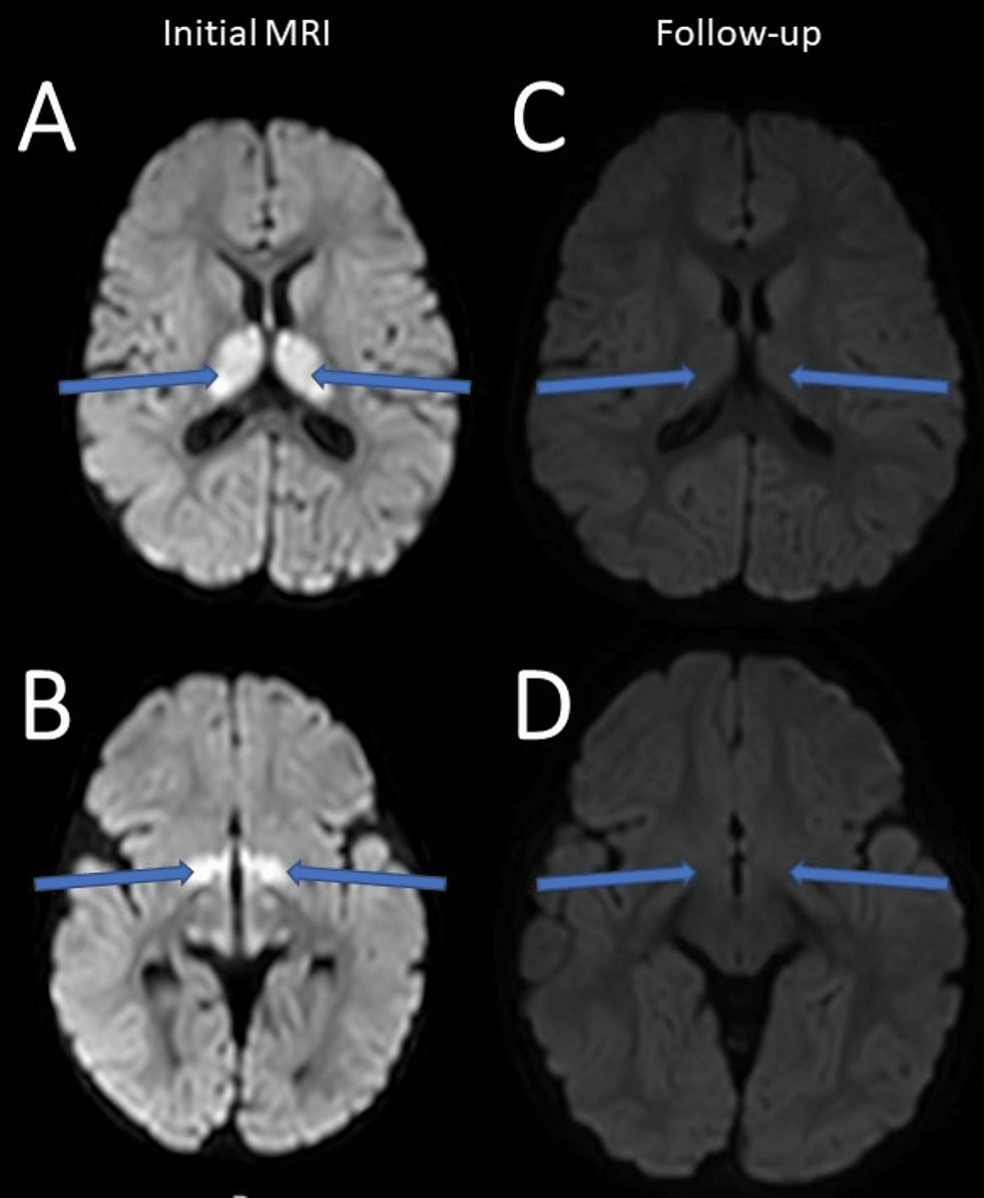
Vigabatrin-induced transient hyperintensities with diffusion restriction in bilateral (A) thalami and (B) globi pallidi on diffusion-weighted MRI. Follow-up MRI in 17 months showed total resolution of both hyperintensities (C-D).
